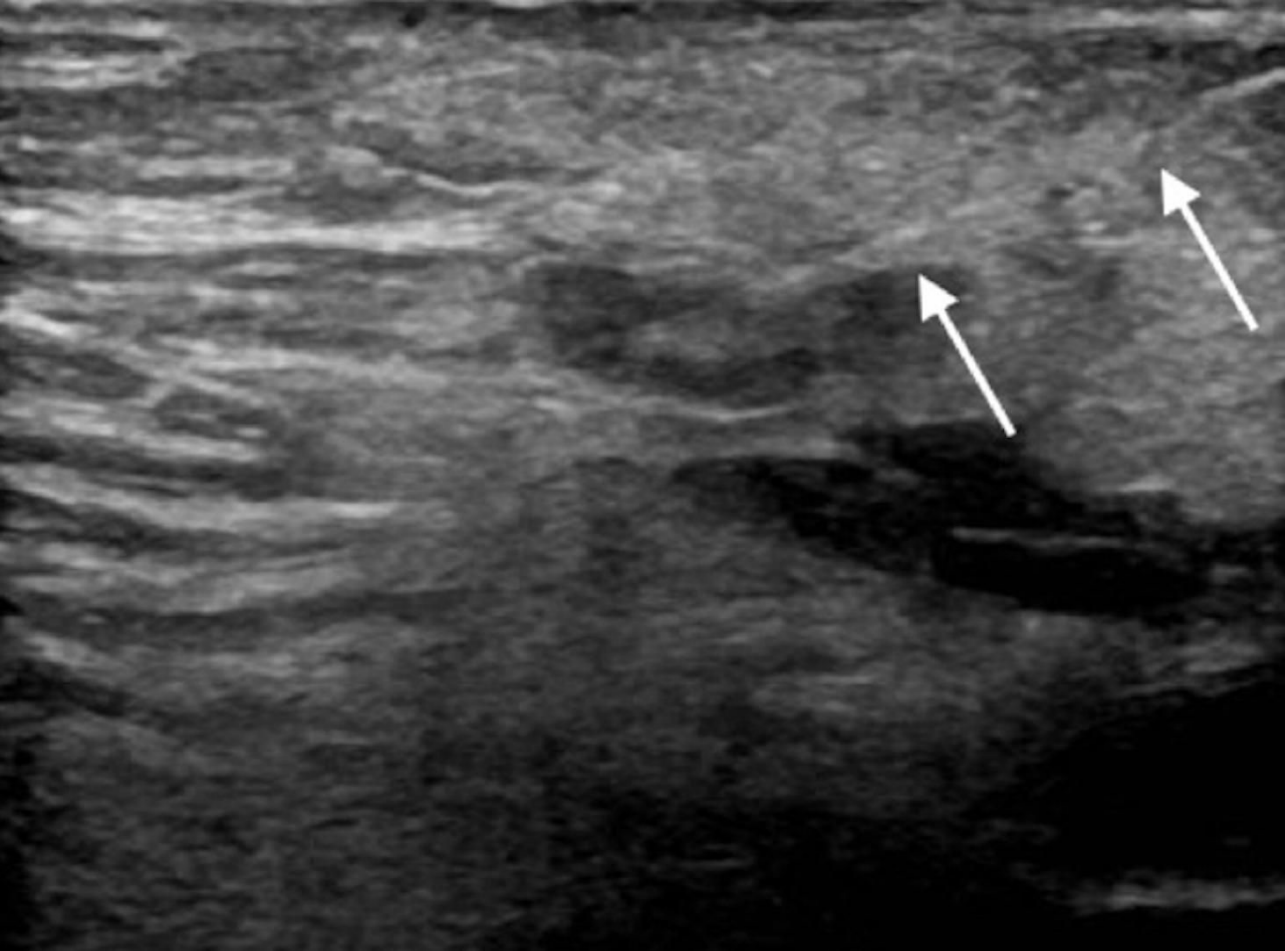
Groin lymph node puncture using a 25-gauge needle (white arrow) under ultrasound guidance.
